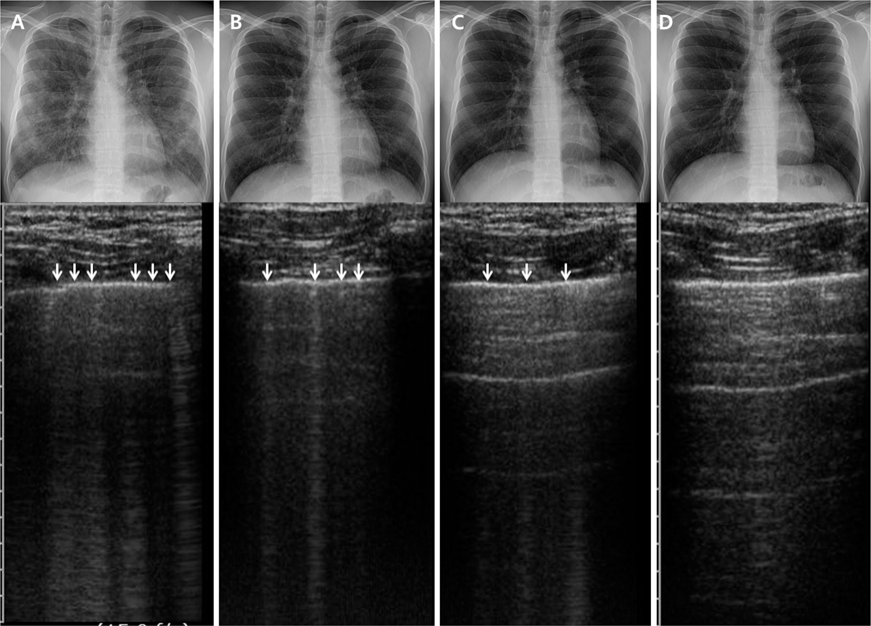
Serial changes in chest radiographic and thoracic ultrasound findings during hospital stays. (A) day 1; (B) day 4; (C) day 7; (D) day 14 (white arrows = B-lines).
